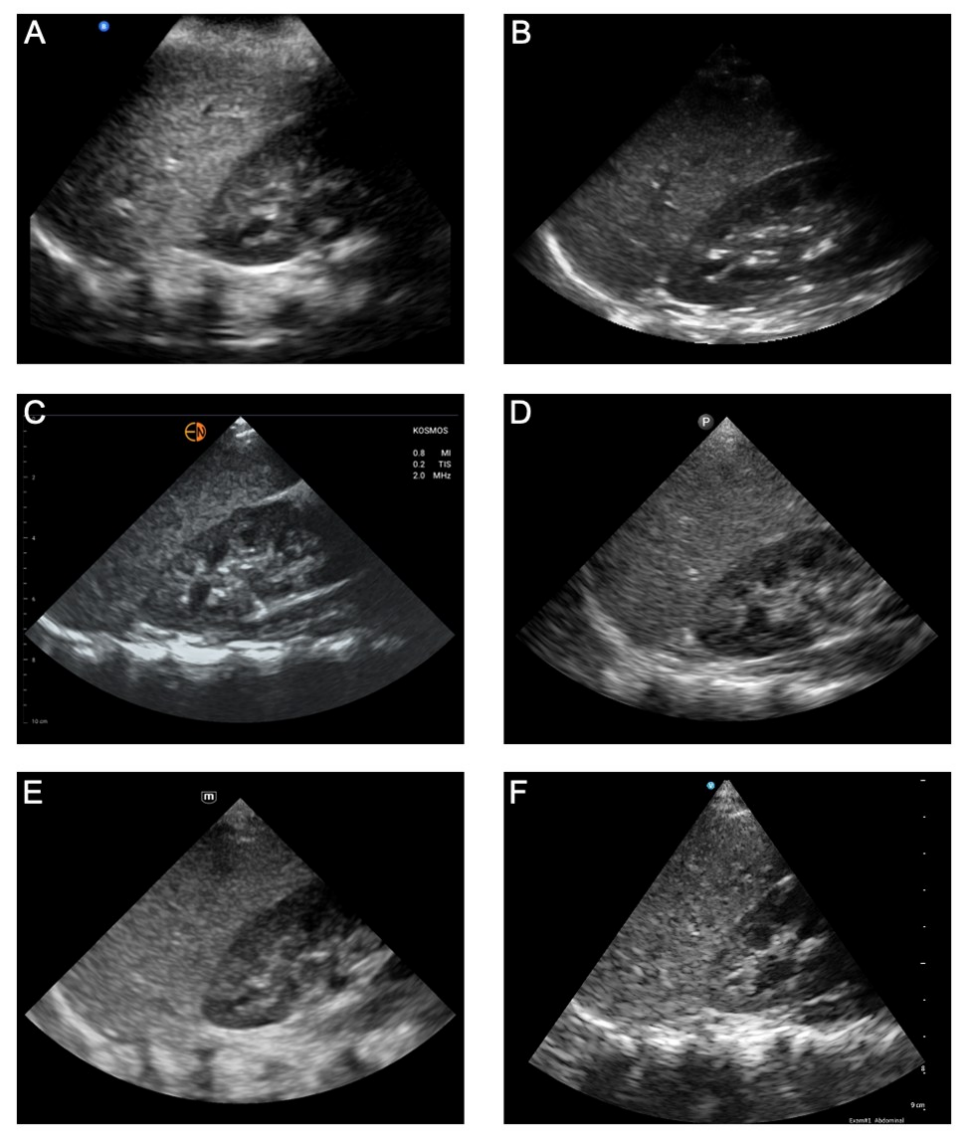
Abdominal Right Upper Quadrant Views acquired from the same pediatric model using six handheld ultrasound devices: (A) Butterfly iQ+™, (B) Clarius®, (C) Kosmos™, (D) Lumify™, (E) Mindray®, and (F) Vscan Air™.
